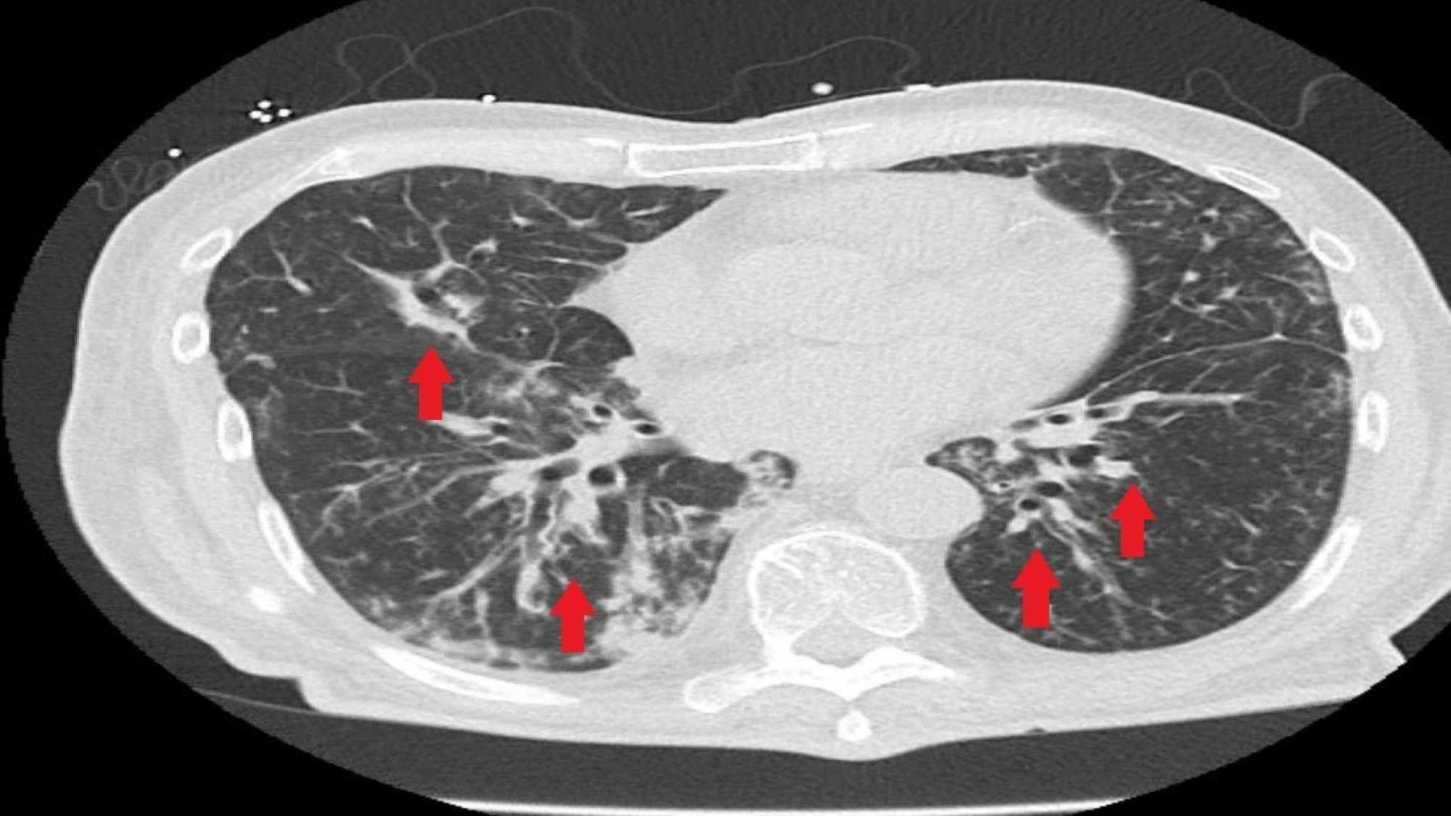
CT chest revealing multifocal densities and bronchiectasis.
